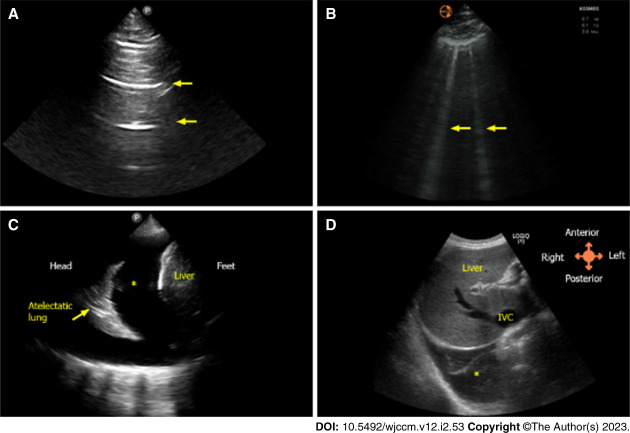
Ultrasound images. (A) Normal lung showing horizontal artifacts (A-line represented by arrows. (B) Vertical artifacts typically seen in congestion (B-line represented by arrows). (C) Pleural effusion indicated by asterisk. (D) IVC. IVC, inferior vena cava. Images used with permission.
